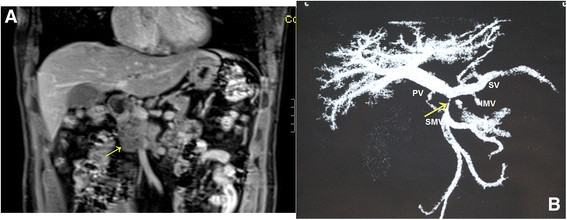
Magnetic resonance imaging (MRI) of a patient with segmental vein occlusion. A) A mass with complete occlusion of the superior mesenteric vein (SMV) (arrow); B) A multiple planar volume reconstruction image showed complete occlusion of the SMV with a venous collateral (arrow); the inferior mesenteric vein (IMV) inserted into the splenic vein (SV). PV, portal vein.
